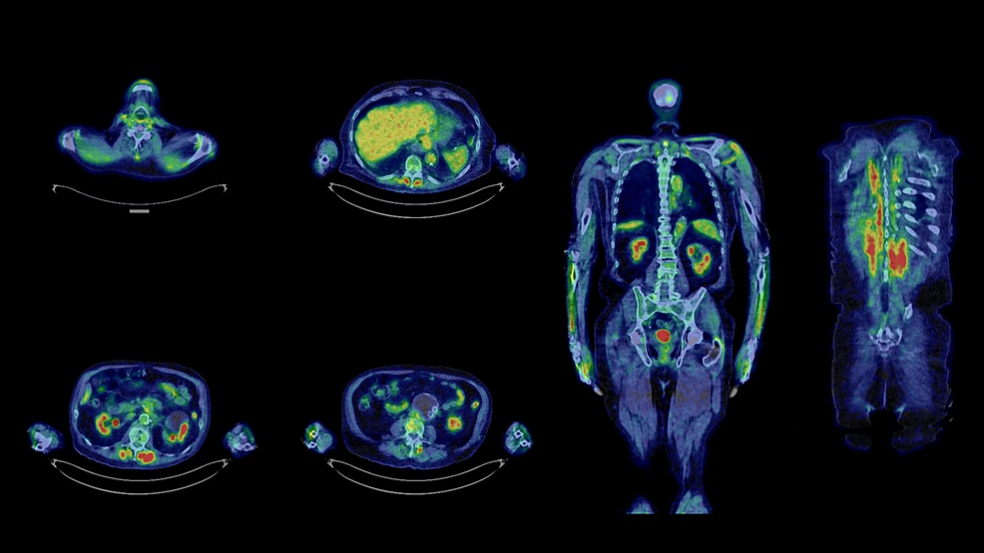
PET/CT scan after CyberKnife treatment Left: axial views. Right: coronal views FDG uptake is not observed in the throat. FDG uptake in the liver, kidneys, intestine, bladder, back muscles, and forearms was considered to be physiological. PET: positron emission tomography; FDG: fluorodeoxyglucose
